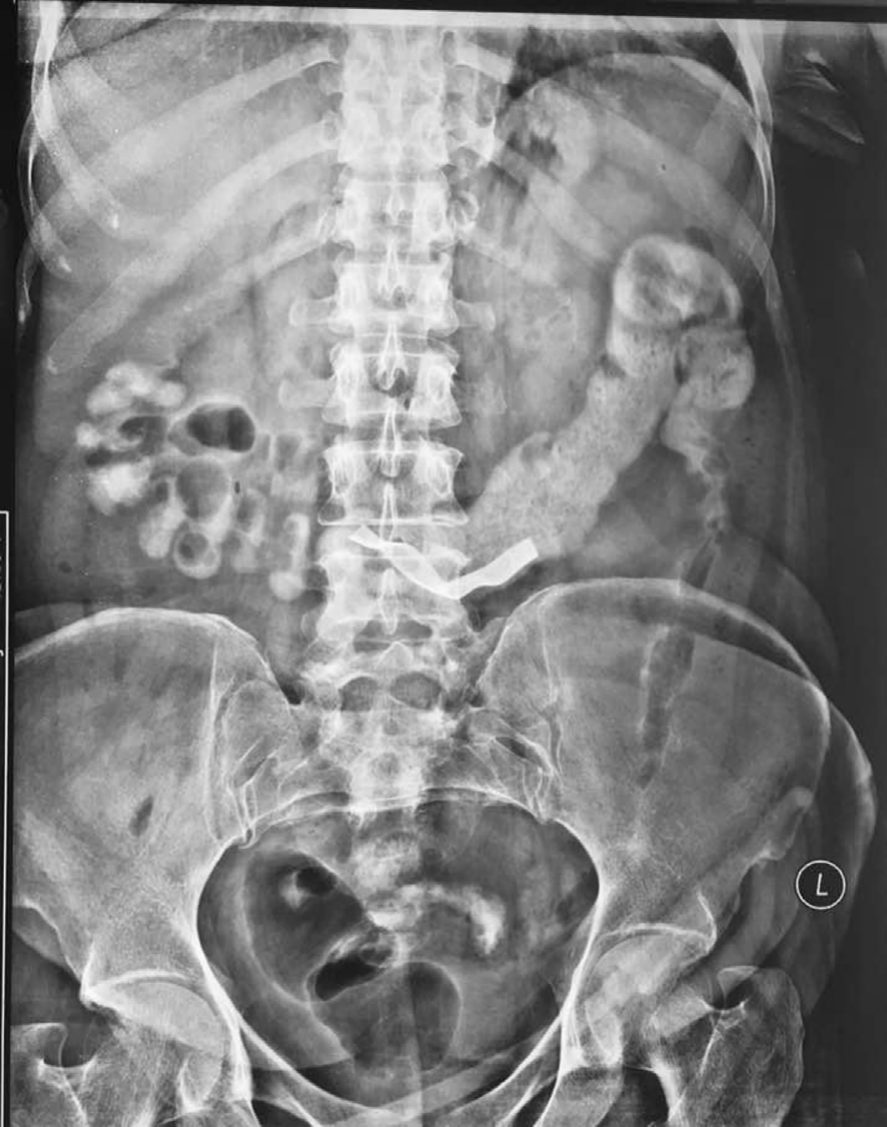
X-ray at 5 hour showing gauze in transverse colon.
